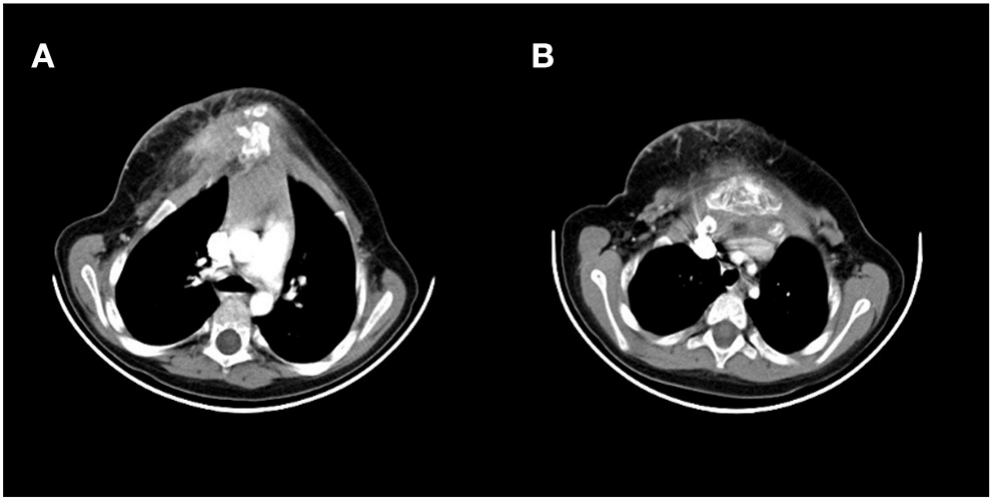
Preoperative CT scan showing a large sternum mass. (A) Uppers segments, (B) lower segments.
